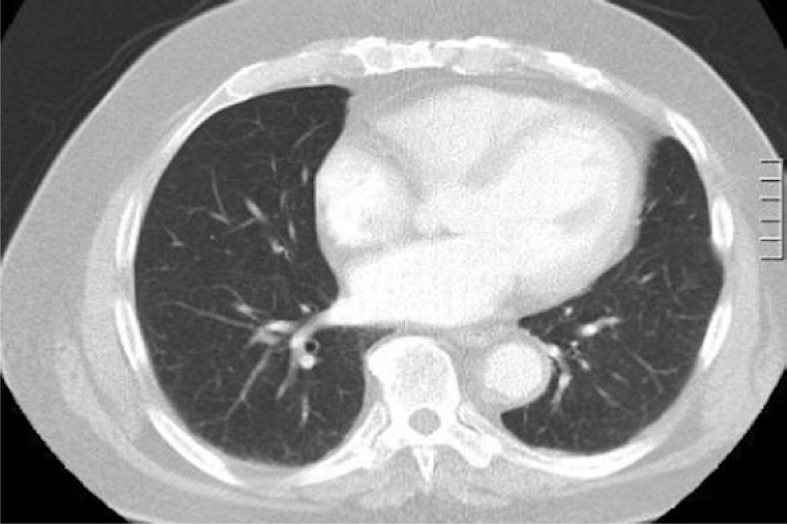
Representative image from initial staging CT; minor emphysematous changes
